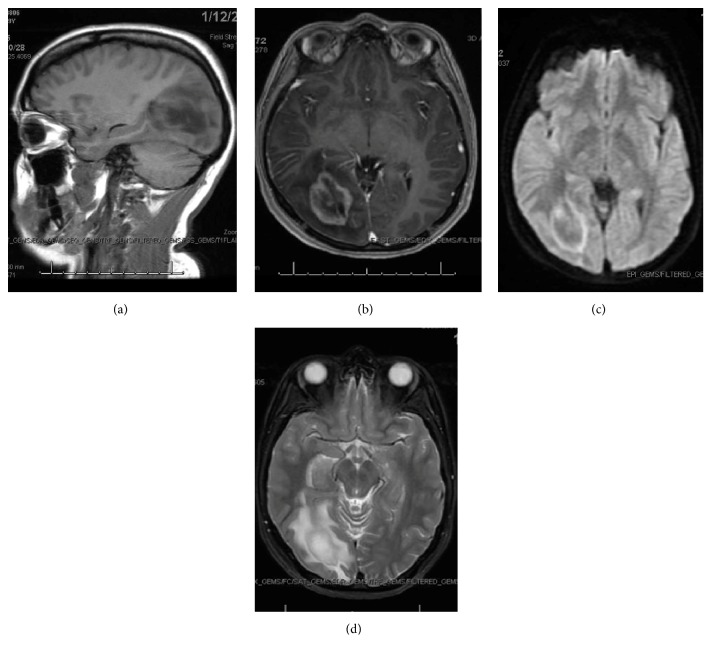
(a) MRI sagittal view T1 FLAIR image. (b) MRI axial T1 BRAVO image. (c) MRI axial DWI image. (d) Axial T2 FSE image. MRI Interpretation: Restricted diffusion in the right occipital lobe. There is compression of the midportion of the occipital horn of the right lateral ventricle. There is enhancement of a presumed mass surrounding the occipital horn of the right lateral ventricle with adjacent vasogenic edema. The possibility that this represents a mass of the right occipital lobe is considered. There is mass effect on the right cerebral hemisphere with effacement of the cortical sulci.
